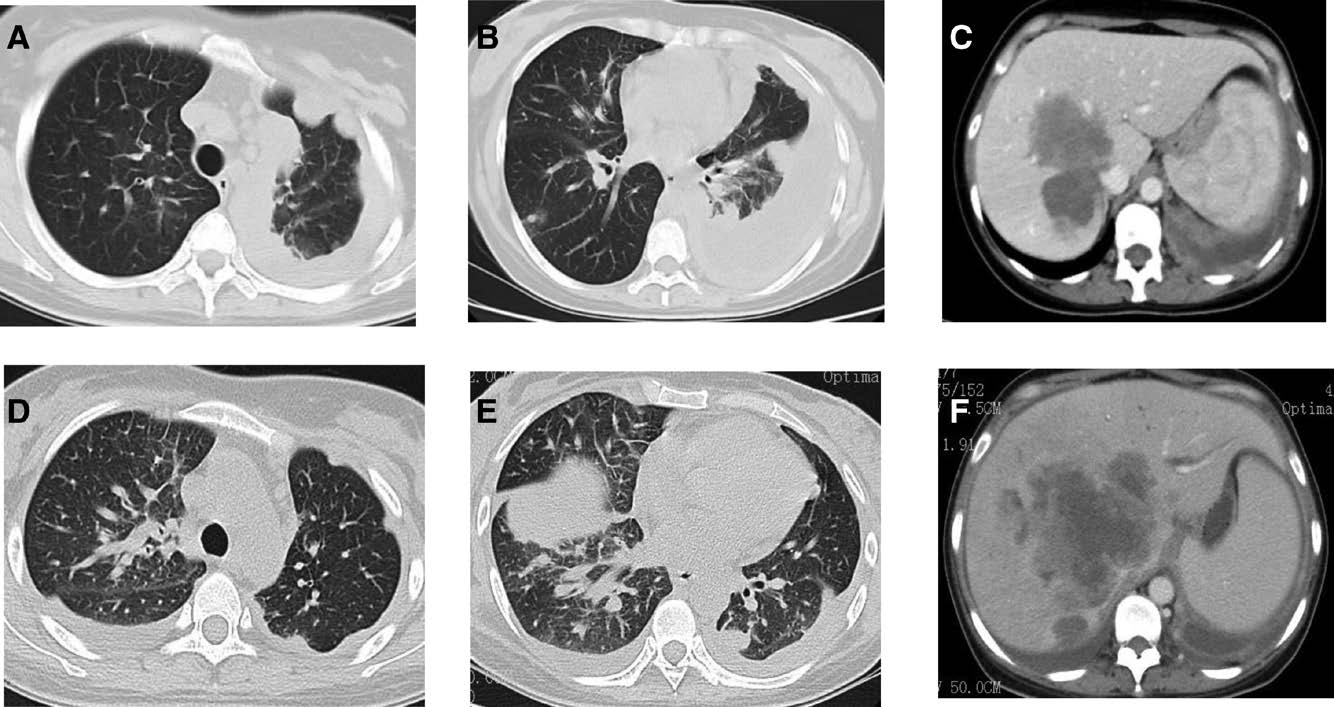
CT scans for (A–C) November 2021 and (D–F) January 2022. CT = computed tomography.
